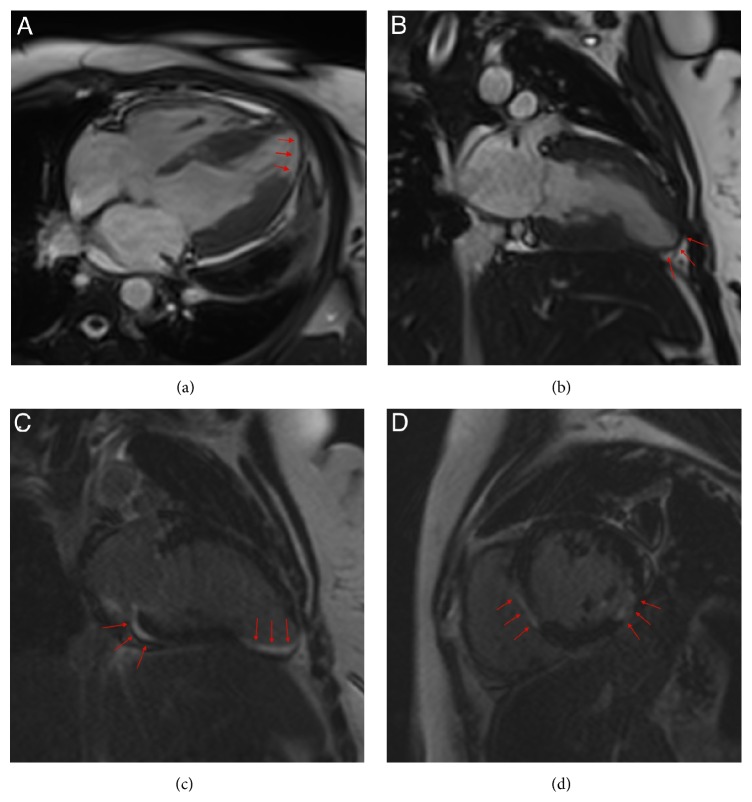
MRI scan with apical thinning of the myocardium (arrows) in 4-chamber view (a) and 2-chamber view (b). Late gadolinium enhancement (arrows) of the apical and inferobasal segments in 2-chamber view (c) and of the inferolateral and septal segments in short axis view (d).
